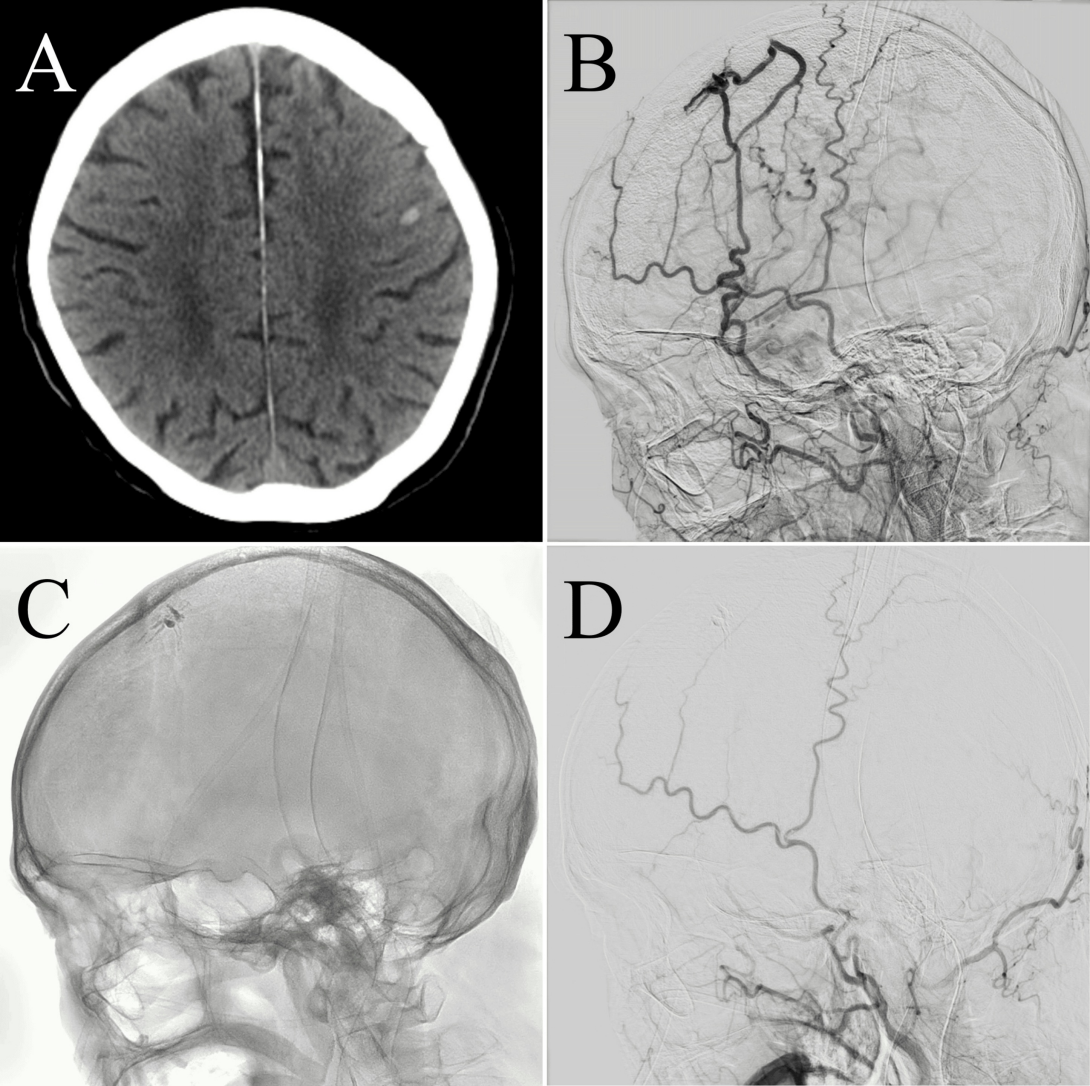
Post-admission imaging (A) and (B) and treatment course (C) and (D). Computed tomography (CT) obtained the day after admission shows an intracerebral hemorrhage (ICH) in the left frontal lobe (A). Repeat left external carotid angiography (lateral view) reveals a pseudophlebitic pattern with marked worsening of venous congestion compared with the previous day's findings (B). Intraoperative angiography following n-butyl cyanoacrylate (NBCA) injection into the left middle meningeal artery (MMA) is shown (C). Postoperative left external carotid angiography demonstrates near-complete resolution of the dural arteriovenous fistula (dAVF) (D).
